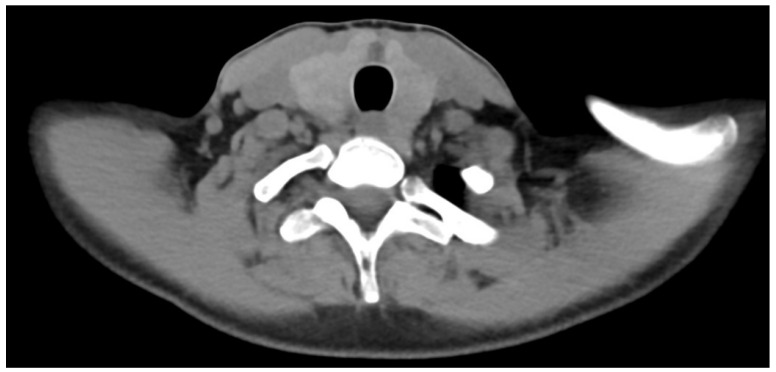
Diagnostic transaxial CT.
